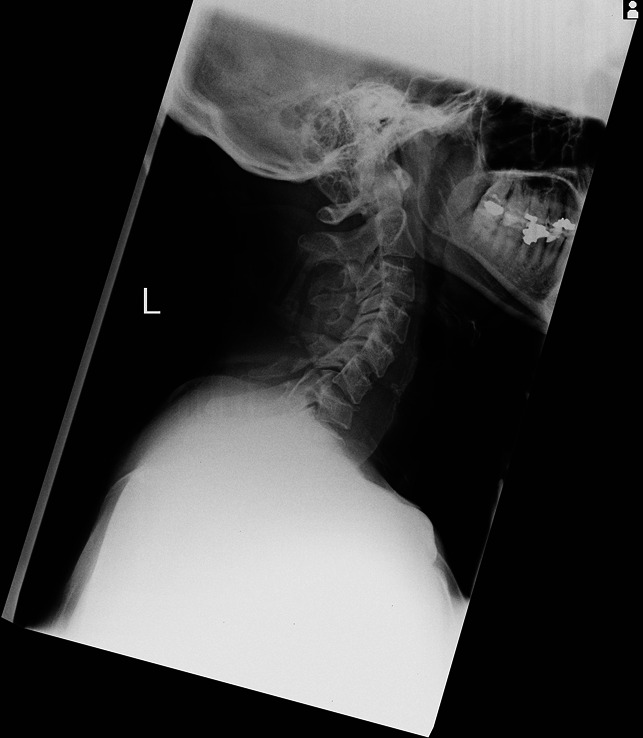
Erect c-spine radiograph showing instability.
